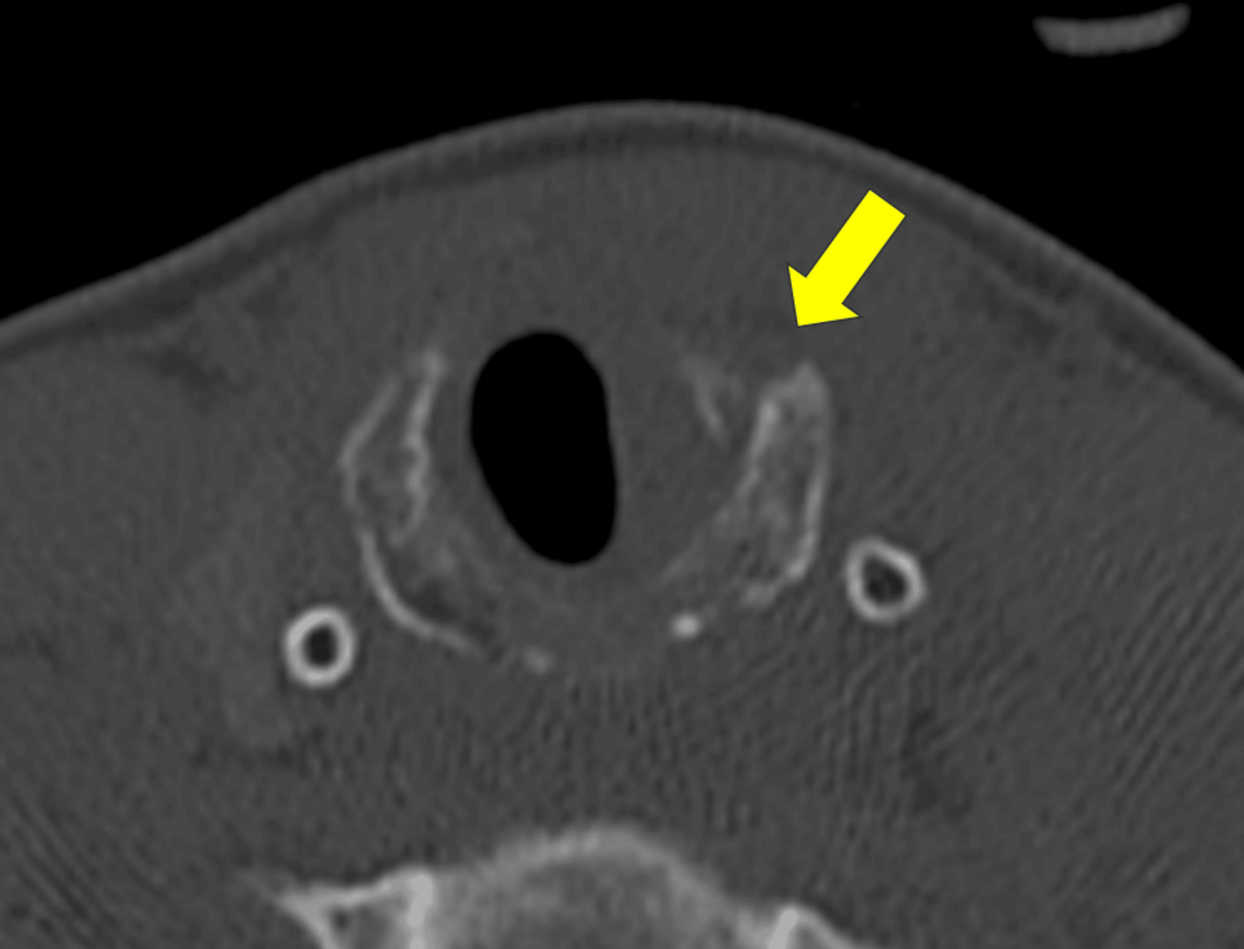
CT of the neck at the emergency department demonstrating a displaced fracture of the left anterior arch of the cricoid cartilage (arrow) with a hematoma.
